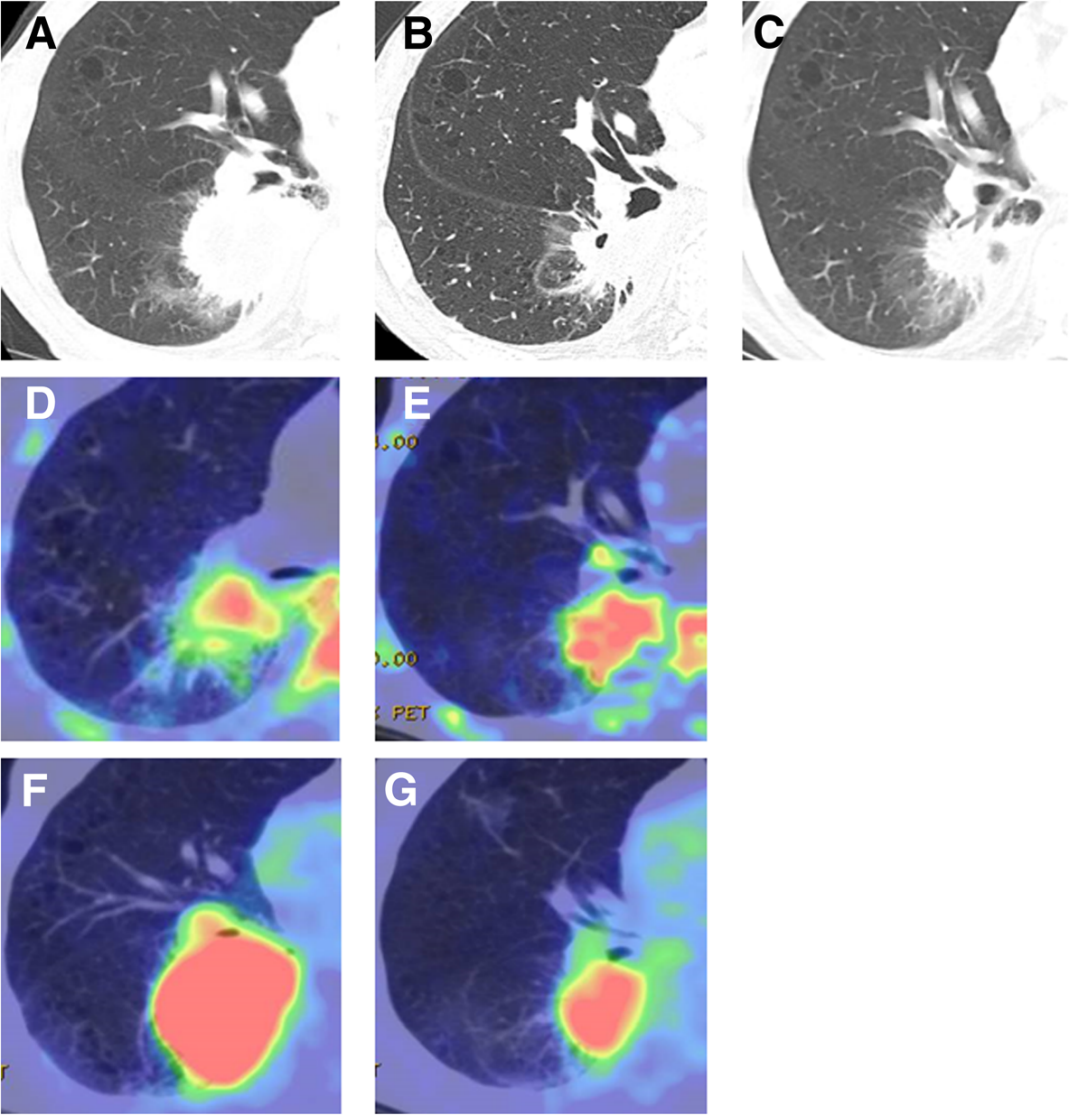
a Chest CT image at baseline. b Chest CT image after 2 cycles of platinum-based doublet chemotherapy (PT-DC). c Chest CT image after 5 cycles of PT-DC. d 4DST PET/CT image at baseline. e 4DST PET/CT image after 2 cycles of PT-DC. f FDG PET/CT image at baseline. g FDG PET/CT image after 2 cycles of PT-DC. Chest CT image at baseline shows lung lesion with a diameter of 55 mm in the right lower lobe (a), with intense uptake of both FDG (f) (SUV_max_ 15.8, MTV 81.0, TLG 672.5) and 4DST (d) (SUV_max_ 4.7, MTV 13.6, TLP 42.1). After 2 cycles of PT-DC, the diameter of lung cancer has decreased to 22 mm (b), categorized as partial response based on RECIST. However, 4DST uptake and uptake area were increased after 2 cycles of PT-DC (e) (SUV_max_ 4.9, MTV 18.2, TLP 56.4) compared to baseline 4DST PET/CT (d). FDG uptake and uptake area were decreased after 2 cycles of PT-DC (g) (SUV_max_ 7.0, MTV 25.0, TLG 86.4), categorized as partial metabolic response based on EORTC and PERCIST. After 5 cycles of PDC, the lung lesion showed regrowth with a diameter of 30 mm (c), indicating recurrence. Recurrence could be predicted earlier with 4DST PET/CT than with chest CT or FDG-PET/CT
